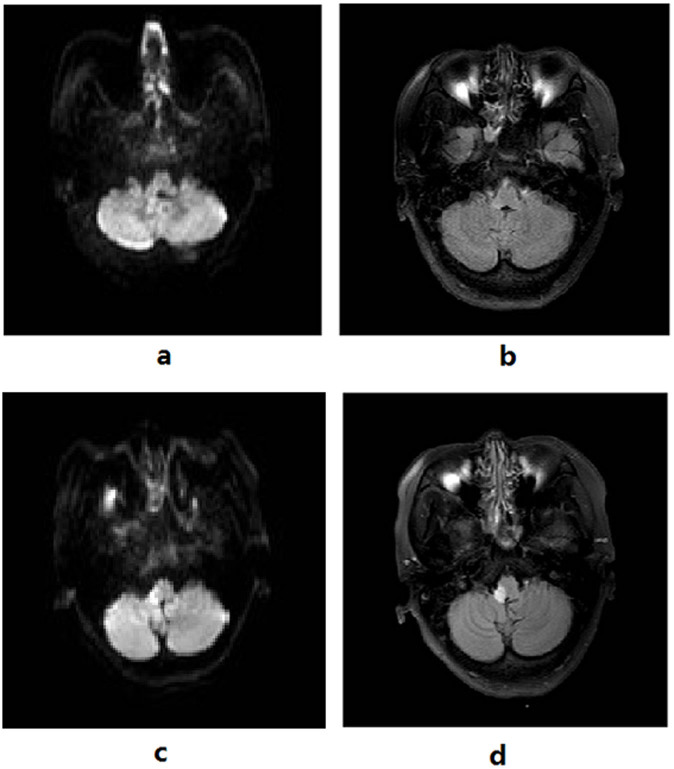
A 44-year old woman with acute cerebral infarction had a NIHSS score of 5 at admission. No acute lesion was seen on MRI DWI at admission and cerebral MRA indicated indistinct visualization of the right vertebral artery. Cervical MRA showed narrowing of the right vertebral artery, stenosis of V2–V5. Cerebral MRI after one week indicated infarction of the right medulla oblongata. Initial cerebral MRI DWI (a) and FLAIR (b); repeat DWI (c) and FLAIR (d).
